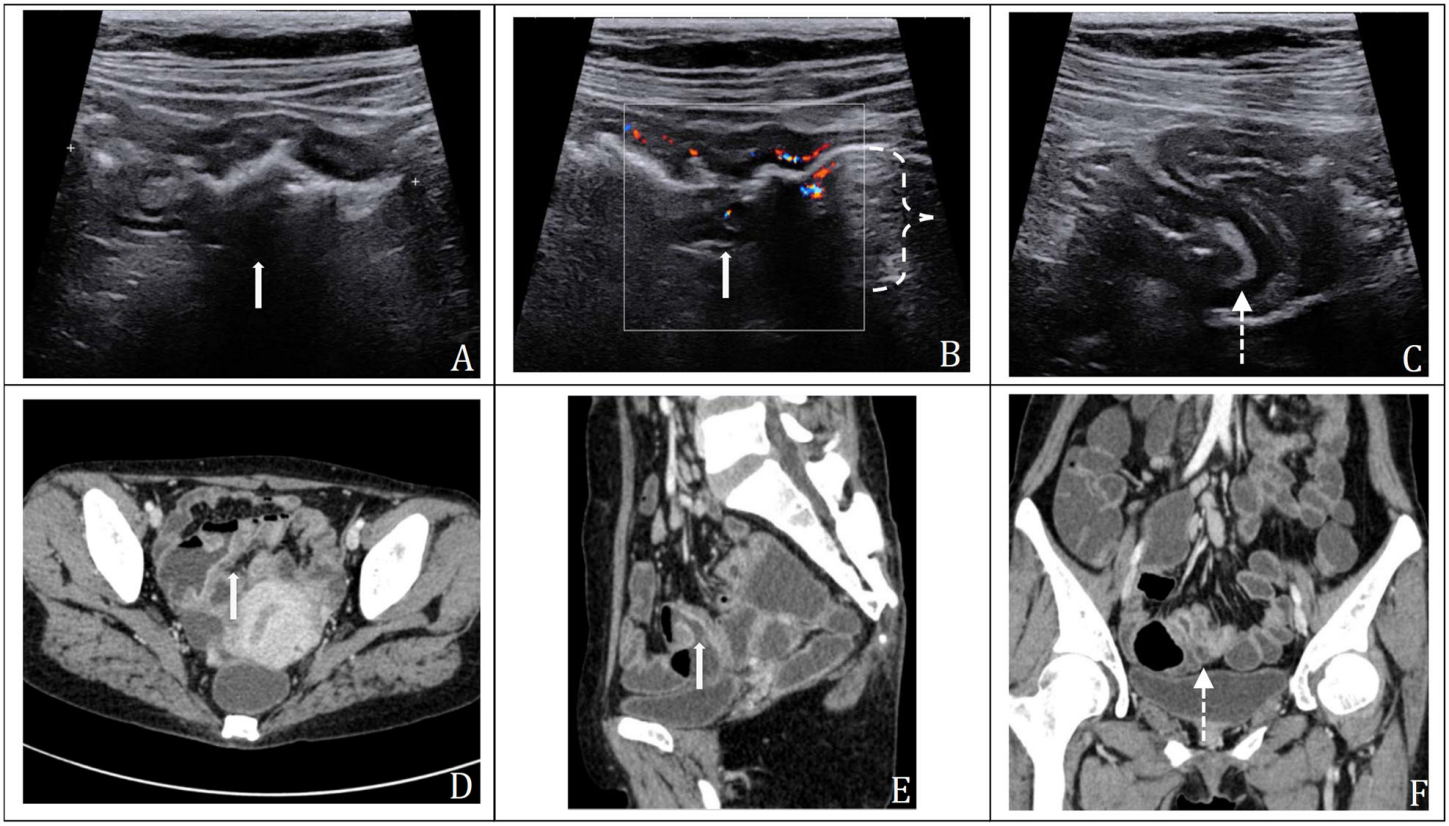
IUS and CTE images of a 41-year-old female diagnosed with CMUSE. The patient was admitted due to melena and intermittent abdominal pain for 2 years. The blood test showed a low hemoglobin count as low as 40 g/L. A, B Longitudinal sonogram of the involved intestine showed a short segmental lesion, bowel wall thickening (0.6 cm) with clear stratification (arrows); B color Doppler ultrasound showed longer stretches vascularity on the bowel wall (Limberg score: III). The proximal small bowel dilation (2.0 cm in diameter, dashed bracket) suggesting the stricture (arrow); C another segmental twisted small intestine with thickened bowel wall (dot-dashed white arrow) in the pelvic cavity was visible; D, E axial plane and reconstructed sagittal plane showing small bowel wall thickening with mural enhancement (arrows), lumen narrowing and proximal intestinal dilation; F reconstructed coronal plane showing another segmental twisted small intestine with thickened bowel wall and mural enhancement (dot-dashed white arrow). CTE confirmed the lesions displayed by IUS
